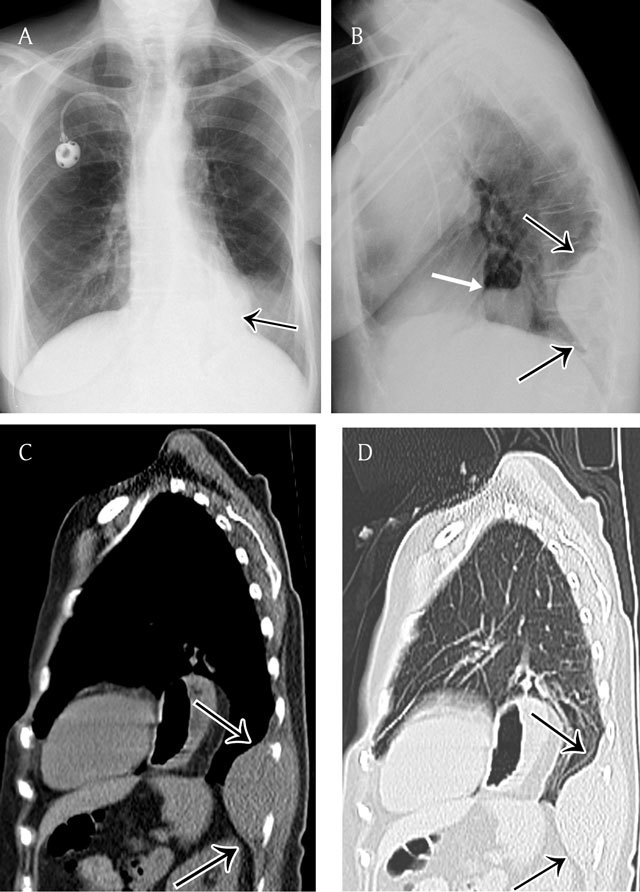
Extrapleural opacity. Hodgkin’s lymphoma of the rib cage – Frontal chest radiograph (a) shows a homogeneous retrocardiac opacity with sharp borders (black arrow). Lateral chest radiograph (b) shows a posterior opacity, presenting with obtuse angles (black arrows). Note the hiatel hernia (white arrow). A sagittal reformatted image in mediastinal (c) and lung window (d) shows the posterior mass and the rib destruction.
